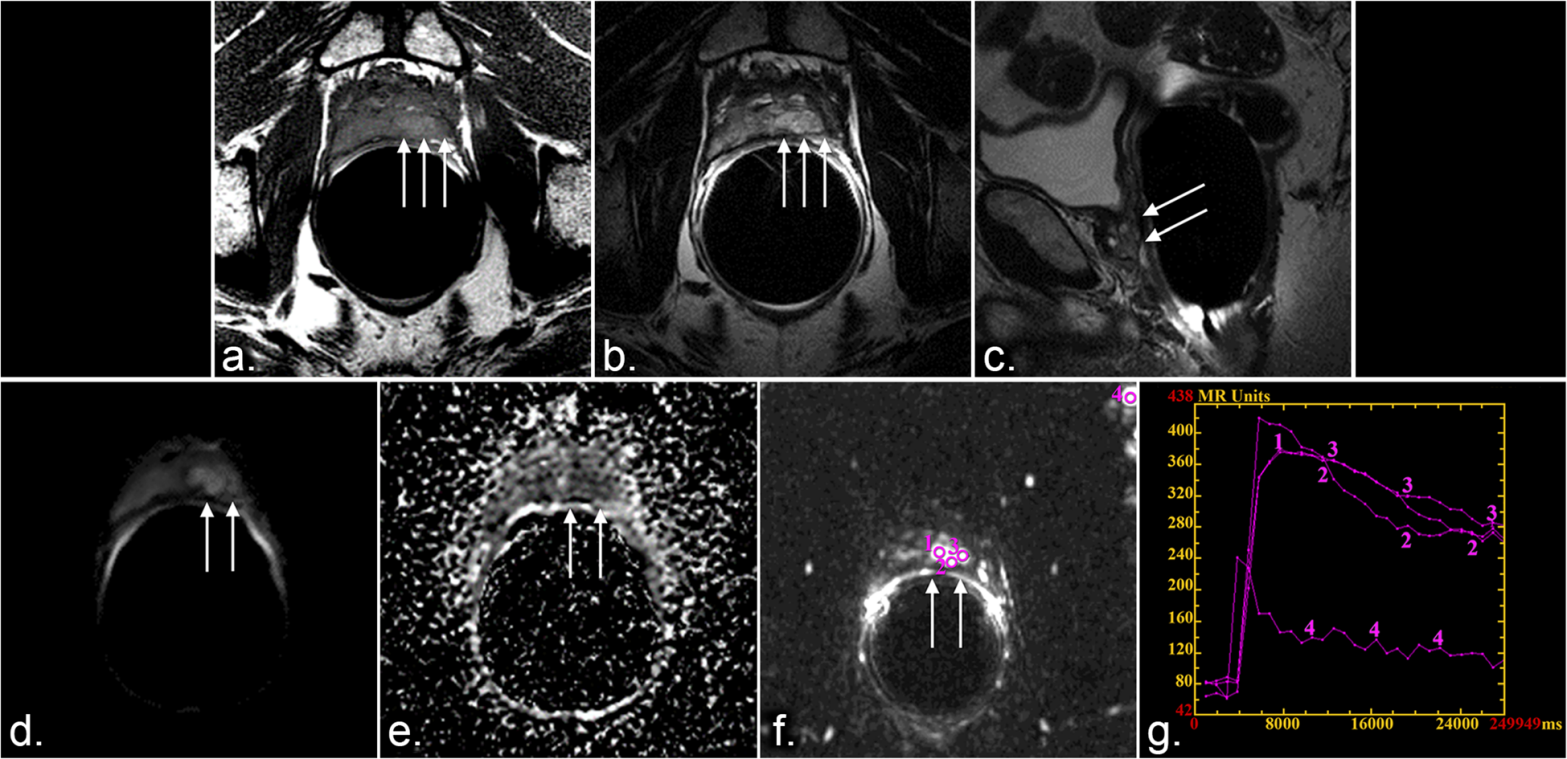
Positive mpMRI in patient with high grade Gleason tumor and elevated PSA (> 1.5 ng/mL) after RP. 69 year old male treated with radical prostatectomy for T3a, Gleason 8 adenocarcinoma presented with BCR after RP (PSA 5.2 ng/mL). Multiparametric MRI including axial T1 (a), axial T2 (b), sagittal T2 (c) axial diffusion weighted images (d), axial apparent diffusion coefficient (ADC) images (e), dynamic contrast enhanced (DCE) MRI (f) images and contrast enhancement time curves (g) reveal confluent T2 hyperintense soft tissue at the level of the vesicourethral anastomosis, consistent with residual prostate tissue. Additionally, an eccentric T1 heterogeneous, T2 hyperintense mass involving the left lateral aspect of the vesicourethral anastomosis is observed (arrows in a, b), extending into the peri-anastomotic soft tissues (arrows in c). The mass is associated with restricted diffusion (arrows in d and e) and focal enhancement (arrows in f). Contrast enhancement time curves (g) demonstrate a pattern of rapid contrast wash in and washout associated with malignancy (curves 1, 2, and 3 = tumor, curve 4 = arterial). Recurrent tumor was confirmed by transurethral biopsy
